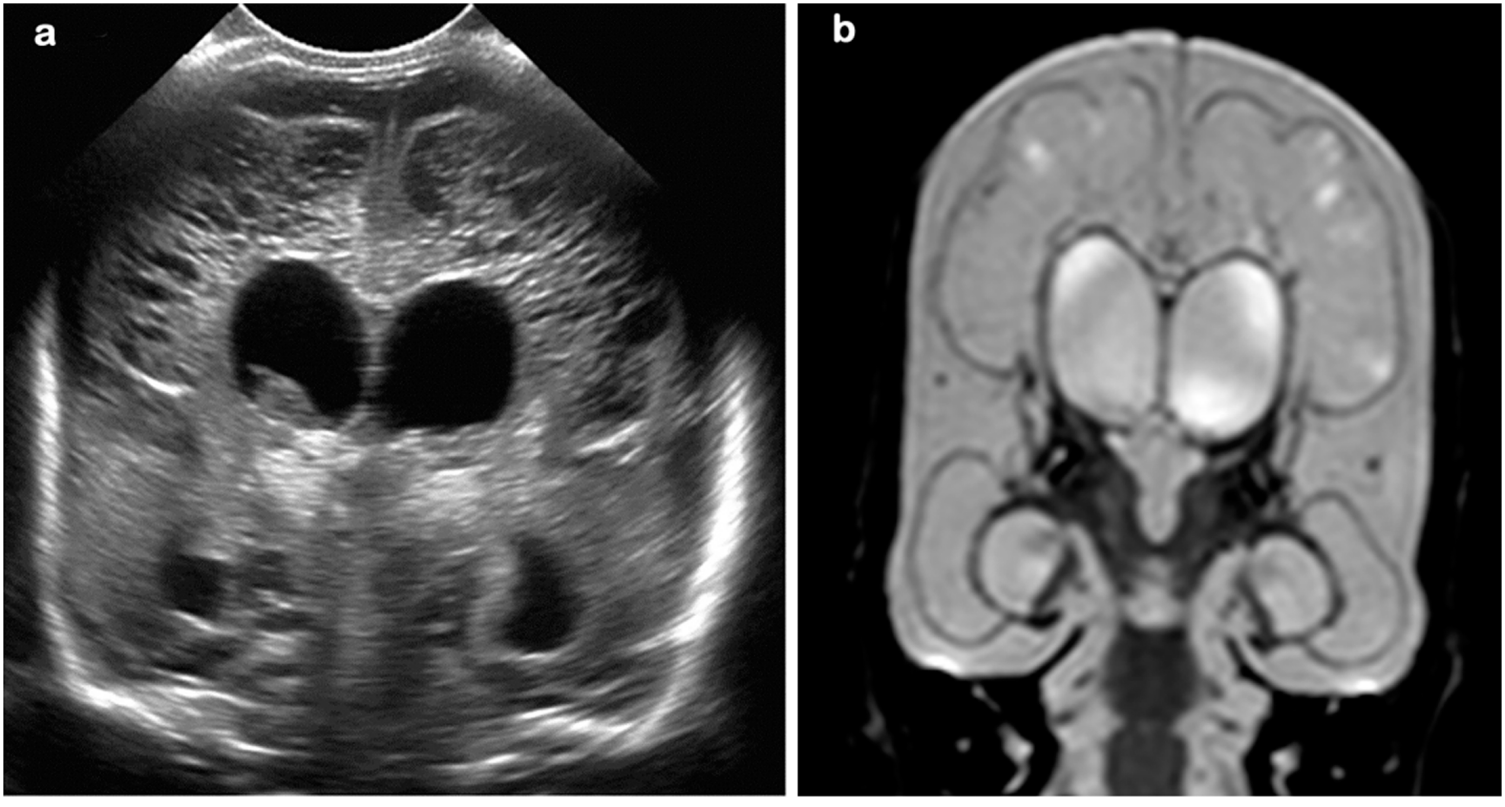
Survivor of monochorionic diamniotic twin pregnancy, who had inhomogeneous echogenicity at birth and showed cystic evolution by day fourteen. Note the discrepancy between the appearance of the cysts on cUS (a) and on the T2-weighted MRI (b), both performed at three weeks. Also note the abnormalities on cUS and MRI in the thalami.
